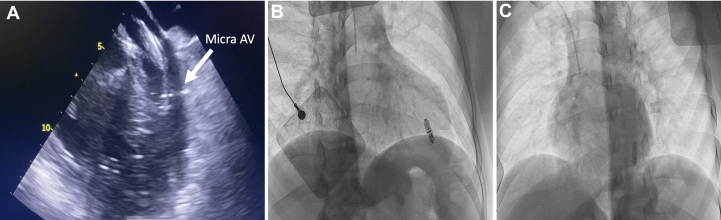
Imaging During and After Micra AV2 Leadless Pacemaker Insertion (A) Transesophageal echocardiography showing the delivery system of the leadless pacemaker crossing the mitral valve. (B and C) Fluoroscopic RAO 33° and LAO 30° views showing the Micra AV2 in the apical septum. LAO = left anterior oblique; RAO = right anterior oblique.
